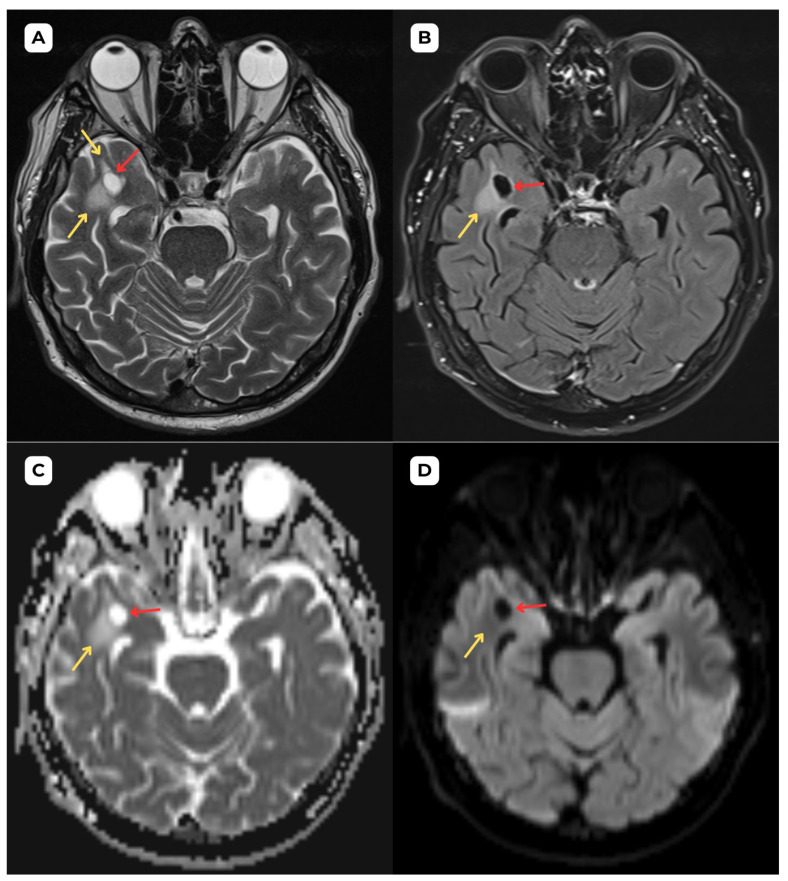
First patient: Brain MRI of a 63-year-old male. (A) Axial T2-weighted sequence. A cystic lesion (red arrow) is seen in the anterior part of the superior temporal gyrus of the right temporal lobe, measuring 1.1 × 0.8 cm, accompanied by perifocal edema (yellow arrows) in the surrounding white matter. (B) Axial T2-FLAIR after contrast image at the corresponding level demonstrates improved visualization of the cerebrospinal fluid (CSF) content of the lesion (red arrow) and highlights the perifocal edema (yellow arrow) more clearly, without contrast enhancement. (C) The ADC map shows a high value with no diffusion restriction on (D) DWI-B1000, where changes show no signs of potential malignancy, most likely benign structural changes. The most likely differential diagnosis is opercular (type IV) perivascular space. Red arrow—opercular (type IV) perivascular space; yellow arrow—surrounding edema. Perivascular spaces of this type (IV) were first clearly documented with MRI imaging in 2020 [1,2], but there is still insufficient documentation of radiological characteristics with vasogenic edema. Type IV PVSs are typically found in the anterior temporal lobe and are closely associated with the middle cerebral artery M2 and M3 segment branches in the white matter of the brain. These PVSs are frequently associated with perifocal edema, likely due to the region’s loose white matter structure and increased interstitial fluid permeability around small penetrating vessels. It should be emphasized that the presence of perifocal edema does not necessarily indicate an active pathological process; it may represent a reactive or structural phenomenon without clinical significance, particularly in elderly individuals [3,4]. Perifocal edema surrounding an opercular perivascular space can range from minimal to very profound and is found in roughly 80% of cases of type IV PVS [5]. In this case, the first patient had more pronounced perifocal edema in both the initial MRI examination (Figure 1 and Figure 2) and the one-year follow-up MRI (Figure 3) than the second patient (Figure 4, Figure 5 and Figure 6).
